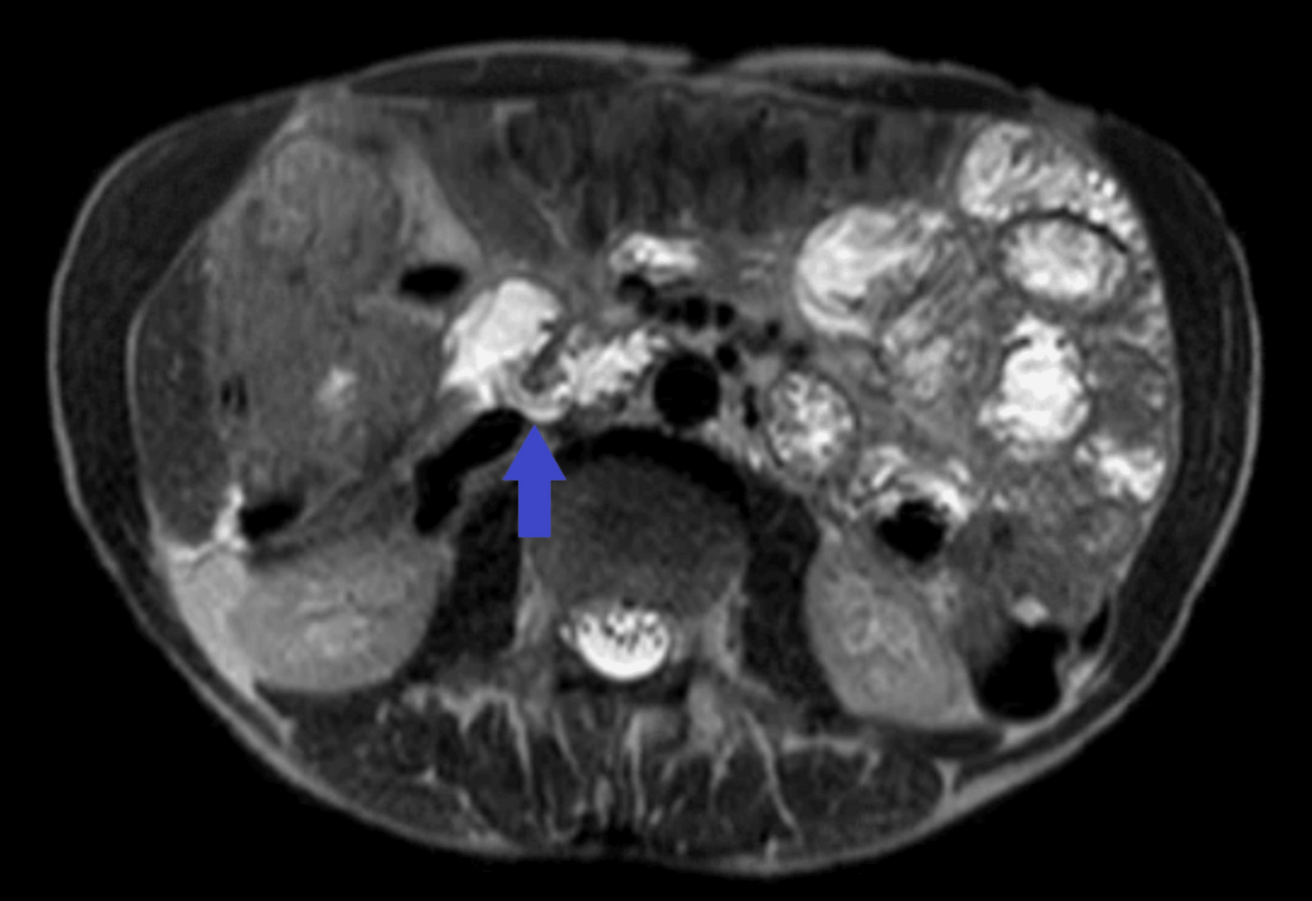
MR Enterography Demonstrating the Fistulous Tract T2-weighted magnetic resonance (MR) enterography image demonstrating the fistulous tract (blue arrow), with fluid content passing from the duodenum to the hepatic flexure of the colon.
